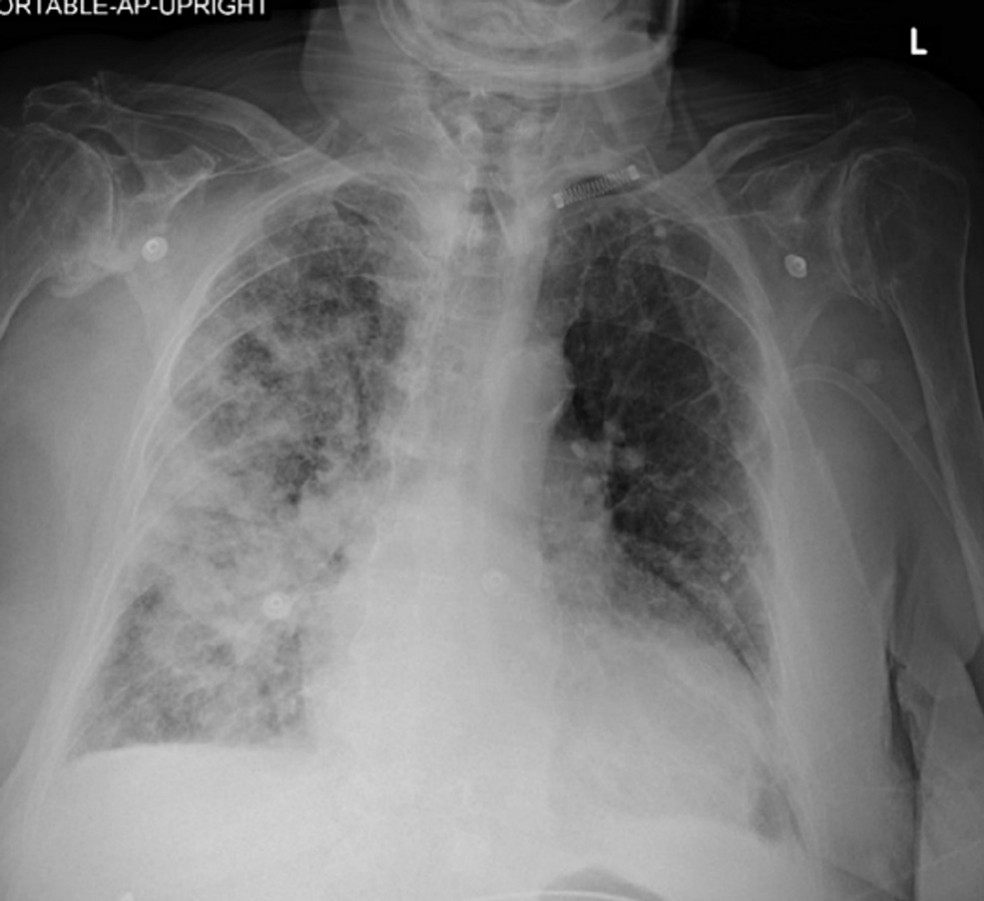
Portable anteroposterior chest X-ray showing alveolar opacity, consolidation, and air bronchogram in the right upper, middle, and lower lung zones. There was significant cardiomegaly. The bilateral costophrenic angles are sharp without any evidence of pleural effusion.
